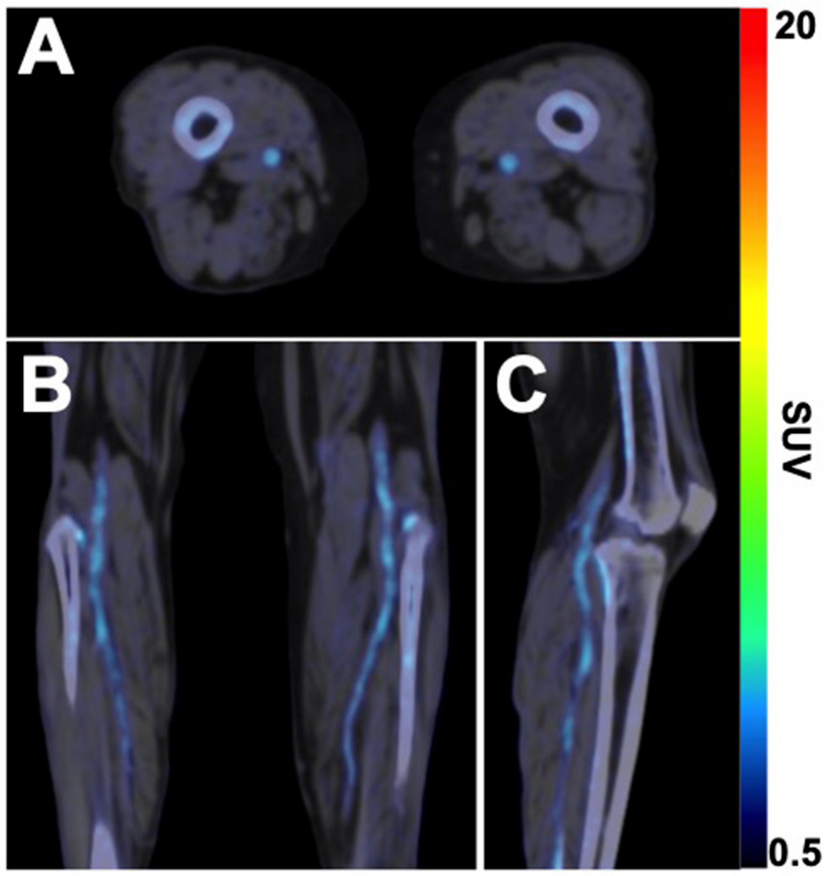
^18^F-NaF PET/CT imaging of active microcalcification in PAD. (A) Axial, (B) coronal, and (C) sagittal fused ^18^F-NaF PET/CT images acquired in a 63-year old female patient with CLTI and type 2 diabetes mellitus demonstrates the active process of microcalcification in above- and below-the-knee arteries. Non-contrast CT images were acquired for attenuation correction of PET data, followed by static PET imaging of the lower extremities 75 min after intravenous administration of ^18^F-NaF (296 MBq). SUV, standardized uptake value.
